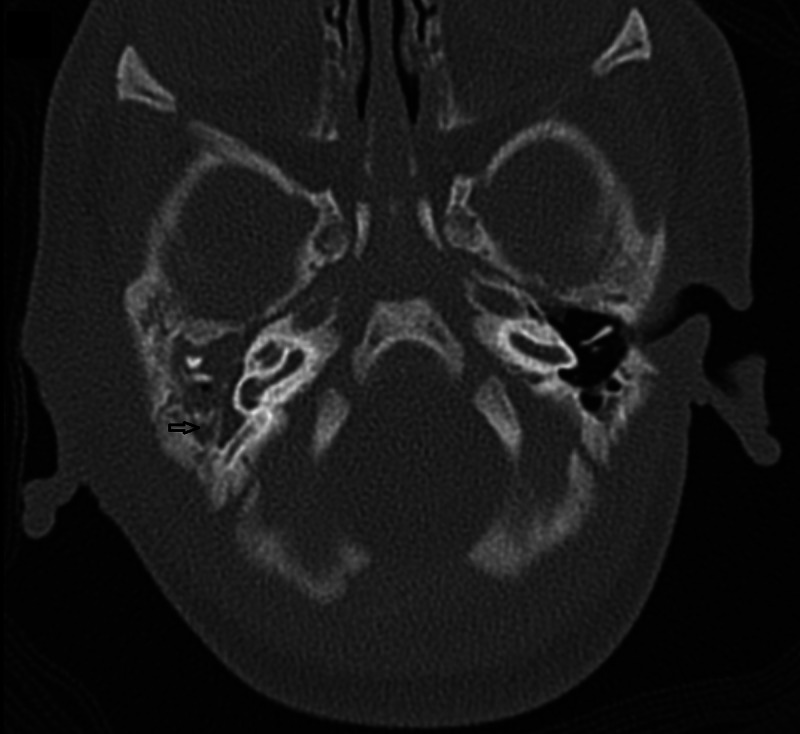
CT of the temporal bone (axial view) showing soft tissue and fluid in the middle ear with fluid-filled right mastoid air cells. The mastoid portion of the facial canal is dehiscent (arrow). The normal configuration of the ossicles could not be visualized. CT, computed tomography
